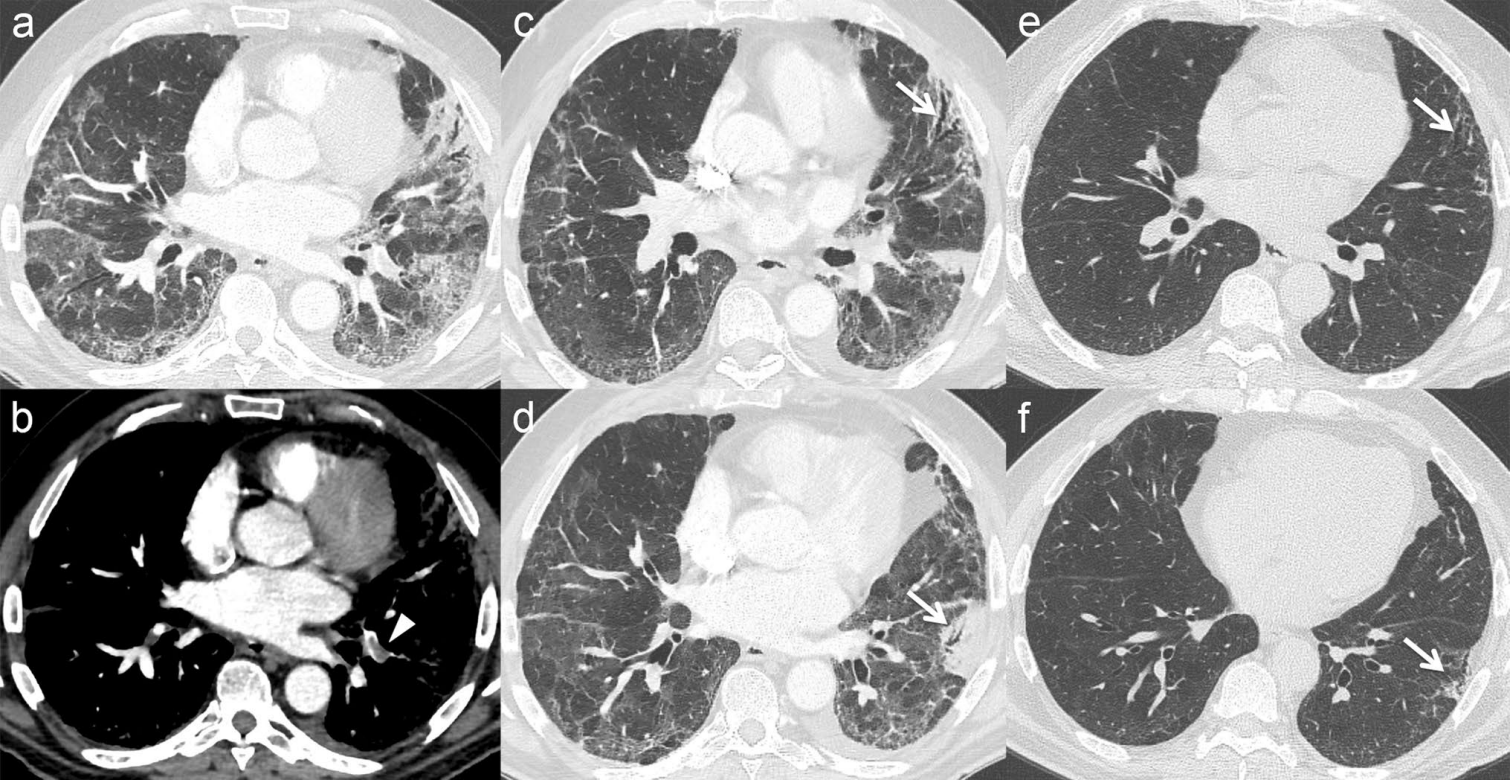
Axial CT scans of a COVID-19 patient with acute pulmonary embolism (arrowhead in b), showing the temporal evolution of the lung abnormalities till the late phase. CT scans in a and b were performed 16 days after the onset of symptoms; CT scans in c and d 30 days after; CT scans in e and f 45 days after. Note the progressive reduction of the areas of consolidation and GG—more extensive in the left lung—and the appearance of fibrotic changes with traction bronchiectasis/bronchiolectasis and irregular interstitial thickening in the lingula (arrows in c and e) and in the left lower lobe (arrow in f), mainly in the area of the previously detected lung infarction (arrow in d)
